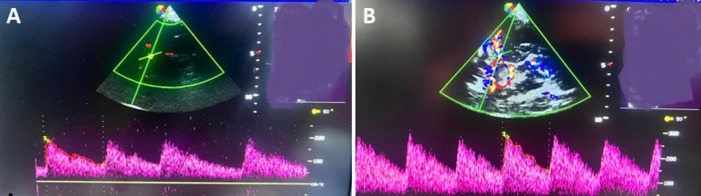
examples of transcranial Doppler ultrasound spectrum: A) left internal carotid artery waveform showing normal velocities with pSV 176 cm/s and TAMMV of 115 cm/s; B) left internal carotid artery waveform showing high PSV 217 cm/s and high intermediate TAMMV 191 cm/s
